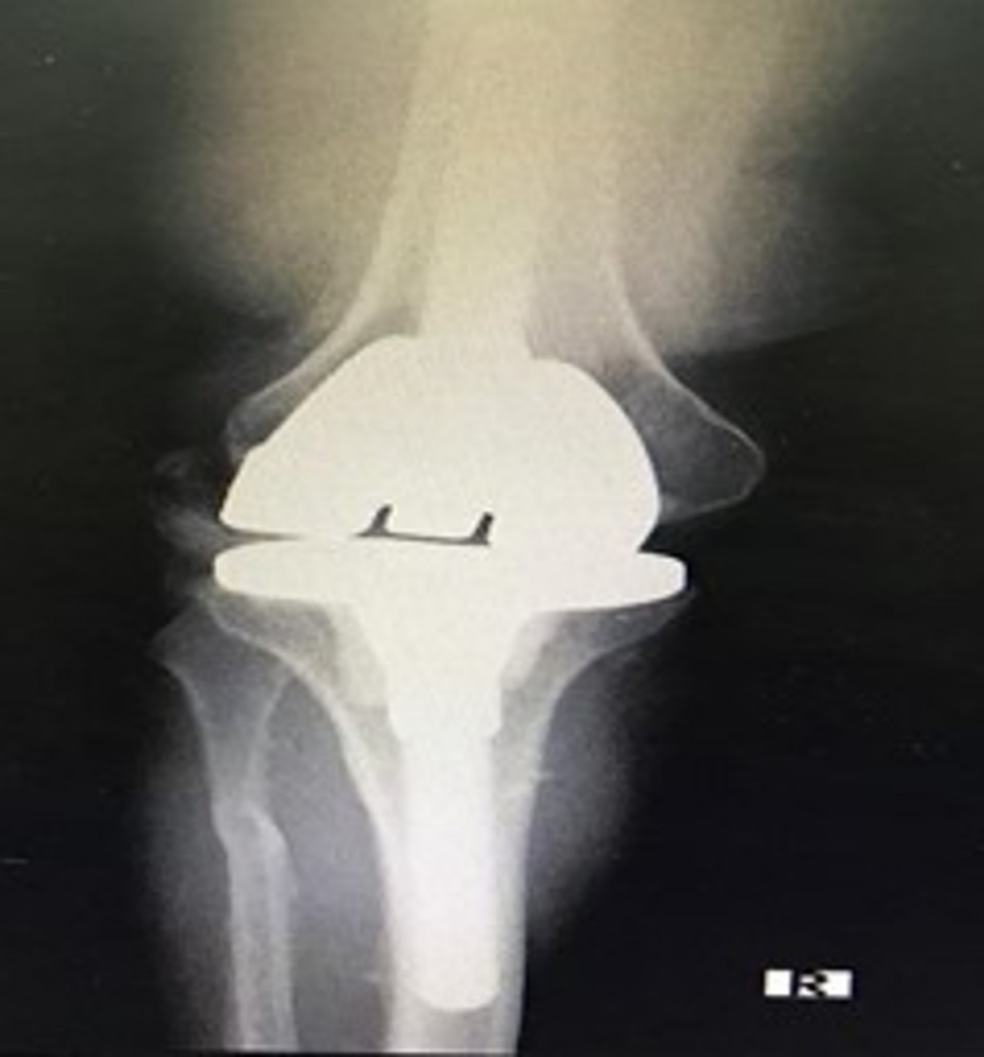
X-ray of the knee post total knee replacement: anteroposterior view.
